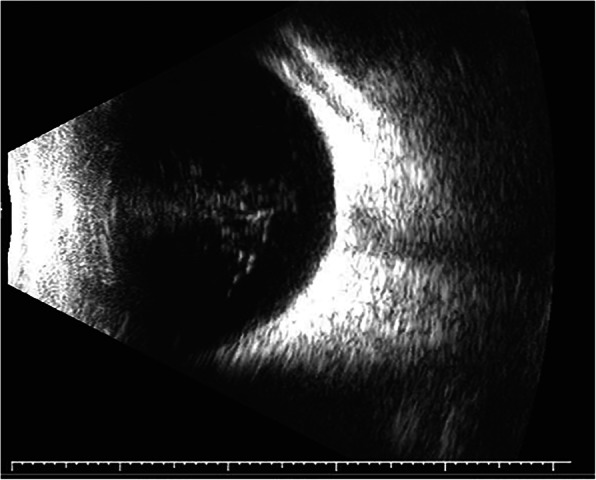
B-mode ultrasound image obtained at the initial visit. The image shows a wide, turbid band extending from the back of the lens to the front of the optic disc. Axis length in the right eye was 26.32 mm
